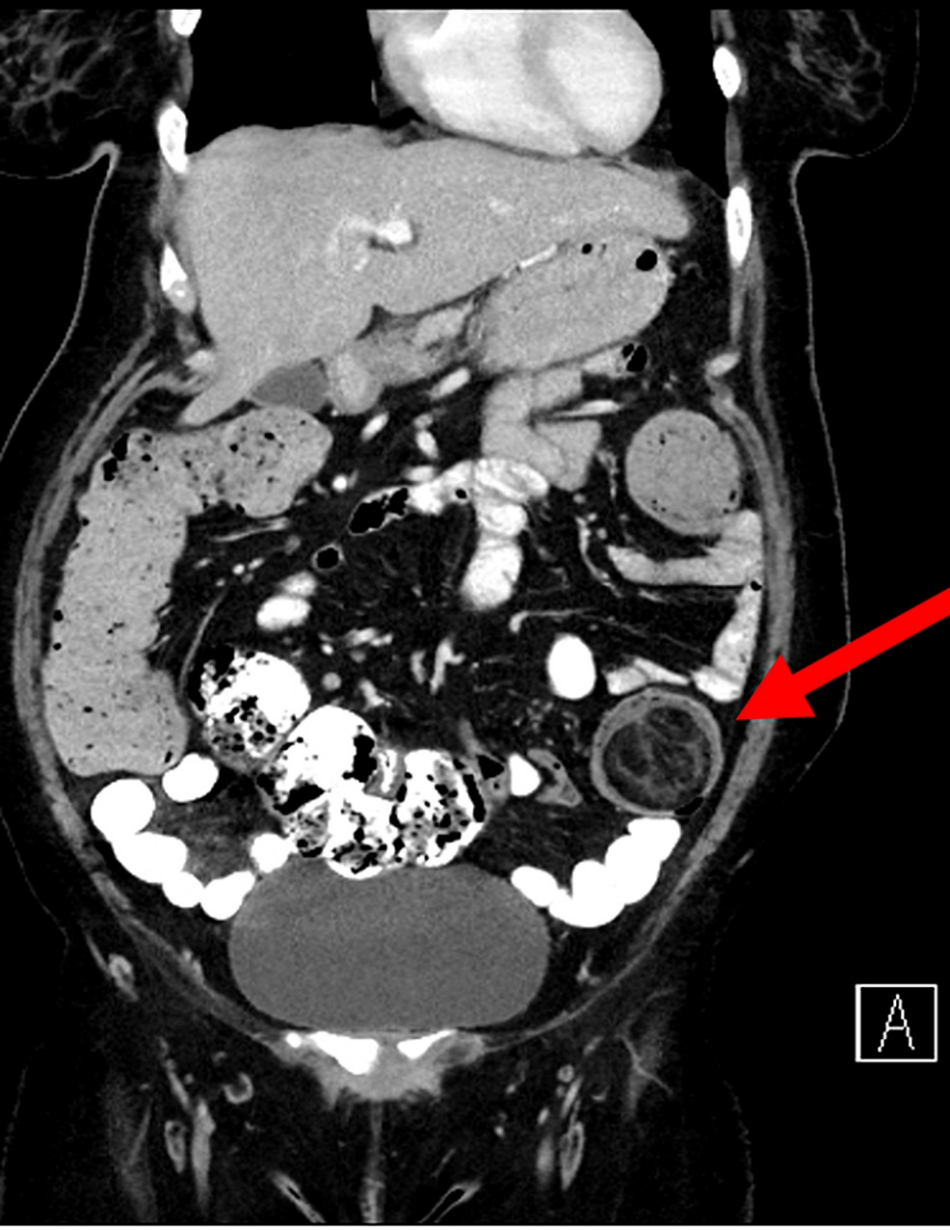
Coronal CT scan showing lipoma
